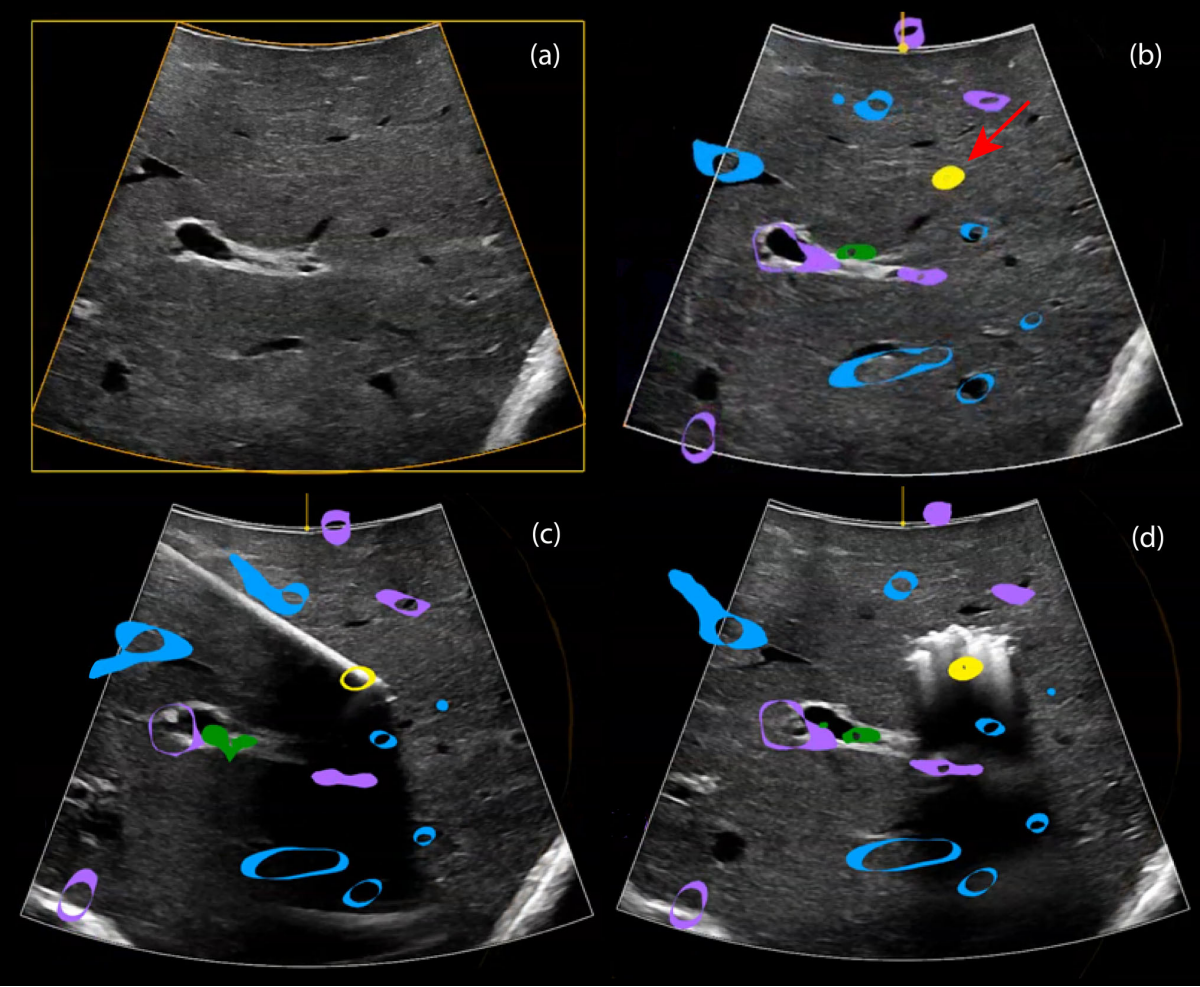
Steps of localization and treatment of a vanished lesion on intraoperative ultrasound using surgical navigation, with a standard ultrasound imaging, b localization of vanished lesion by projection of the patient-specific 3D model on the live ultrasound image, c ablation antenna inserted in vanished lesion based on surgical navigation, and d gas formation after microwave ablation
